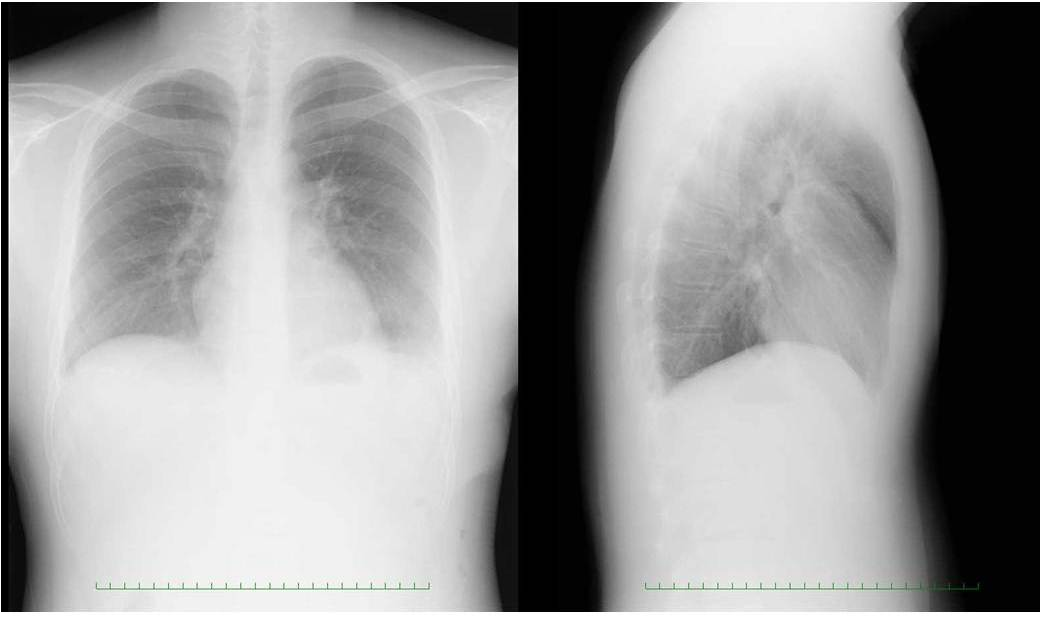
Postoperative chest radiography. There were no abnormalities on postoperative chest radiography.
